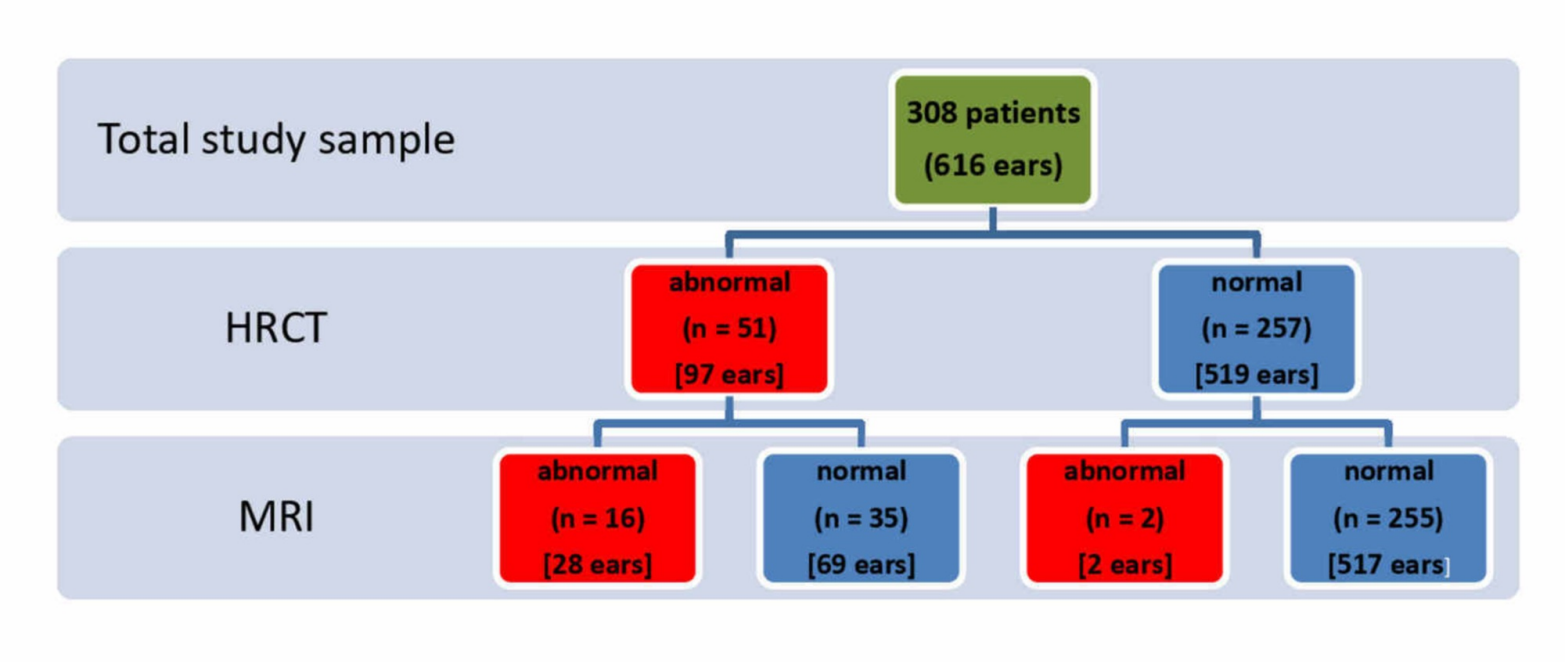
Abnormal findings in radiological examinations conducted before cochlear implantation in a cohort of pediatric and adult subjects HRCT, high-resolution computed tomography; MRI, magnetic resonance imaging
